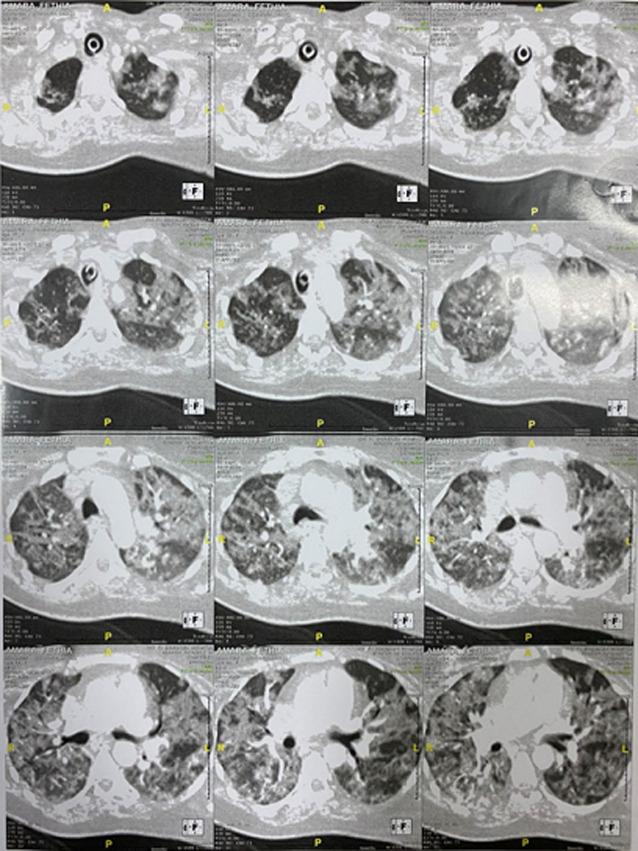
computed tomography pulmonary angiography of the pregnant woman, bilateral diffuse ground-glass opacities, that have a mid and upper zone distribution, reaching more than 75% of the pulmonary parenchyma and associated with subpleural consolidations which predominated in the lung bases and no signs of pulmonary embolism (twelve segments from the same session)
